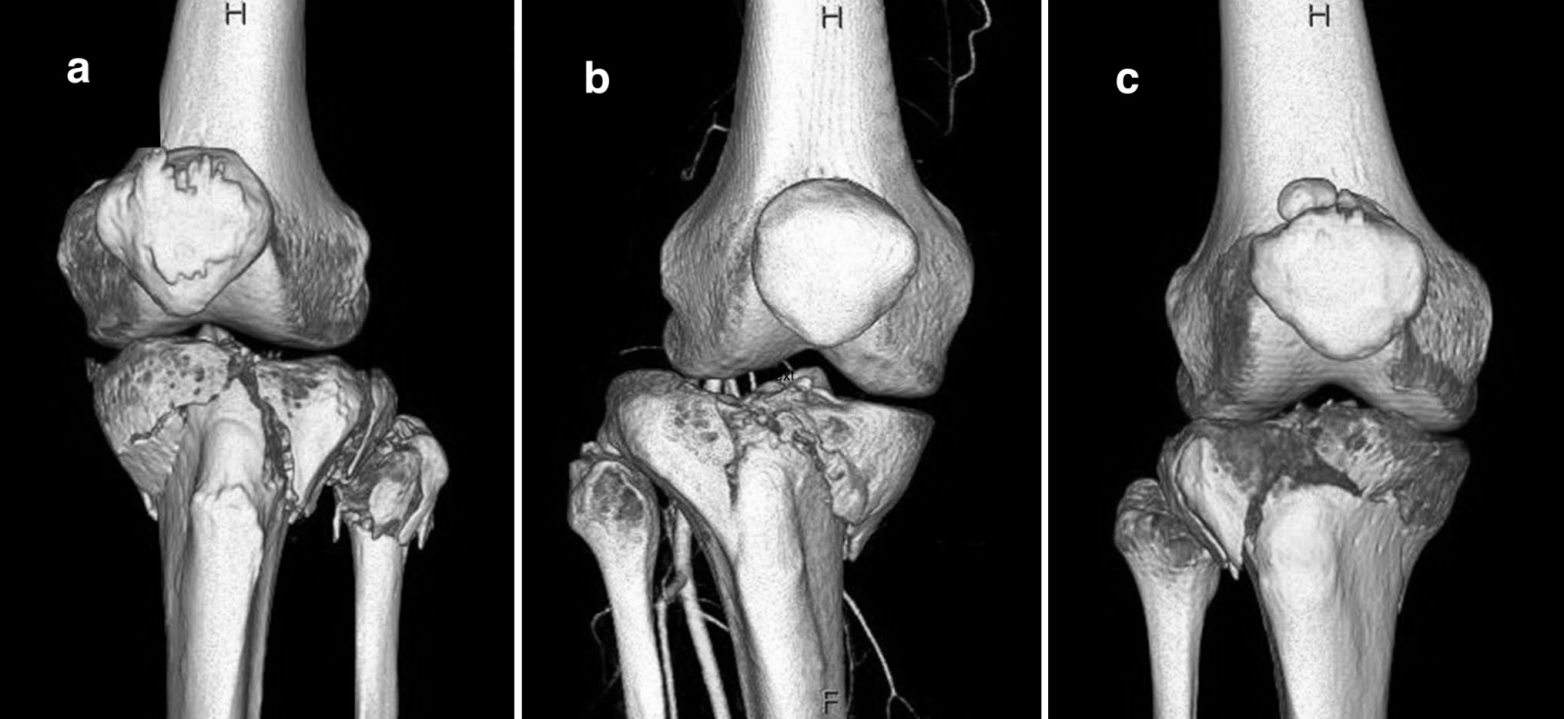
3D CT reconstruction of the three cases a case 1, b case 2, c case 3
